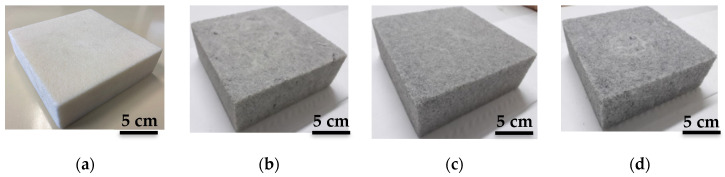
Photos of (a) unloaded epoxy foam and samples loaded with 0.5 wt.% of 3 mm-CFs made with (b) spatula dispersion, (c) shear mixing, and (d) ultrasounds. Mixing duration is 5 min.
